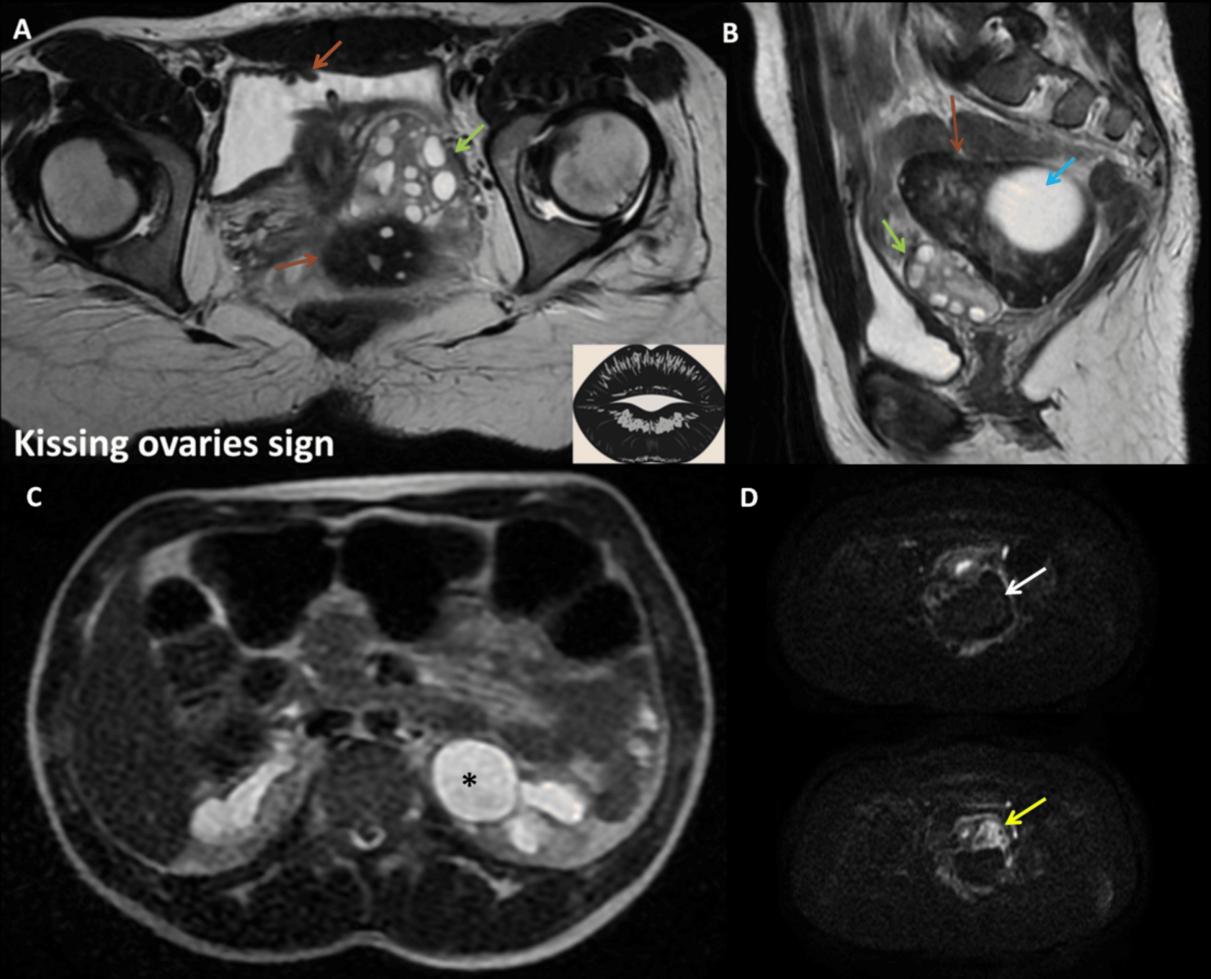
Pelvic MRI assessing the ovary and urinary tract dilation A. Axial T2-weighted MRI demonstrates a normal left ovary containing functional follicles, with a normal isointense stromal signal (green arrow), and a medially displaced right ovary that is markedly hypointense on T2-weighted imaging (red arrow), forming the "kissing ovary sign." Note the normal enterocystoplasty (orange arrow). B. Sagittal T2-weighted MRI shows the enlarged right ovary (red arrow), which retains its reniform morphology with a peripheral hypointense rim and a central heterogeneously hyperintense stroma, reinforcing the suspicion of an edematous stroma. The cystic lesion remains prominent within the right ovary (blue arrow). The left ovary appears normal in size, with multiple follicles and a normal stromal signal (green arrow). C. Axial abdominal T2-weighted imaging demonstrates left pelvic and caliceal dilation (black asterisk). D. Axial diffusion-weighted imaging (DWI) at a b-value of 1200 shows no T2 shine-through hyperintense effect or restricted diffusion within the right ovary (white arrow), while the left ovary exhibits normal diffusion-related hyperintensity (yellow arrow).
